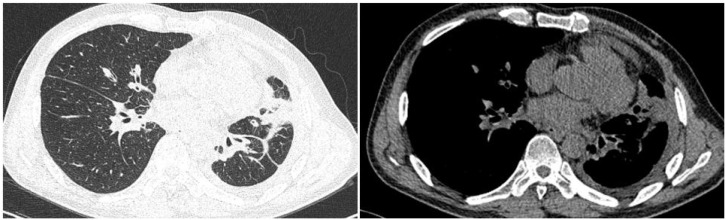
Chest CT scan on March 5, 2024: 1. Considering the medical history, findings consistent with central type lung cancer in the lower lung with pulmonary metastases, along with cancerous lymphangitis in both lungs and left lower lung collapse. There is improvement in mediastinal and left pulmonary hilum lymph node metastases, as well as left pleural metastasis with encapsulating pleural effusion. 2. Multiple solid nodules in both lungs, suggestive of metastatic tumors, showing reduction compared to previous scans.
